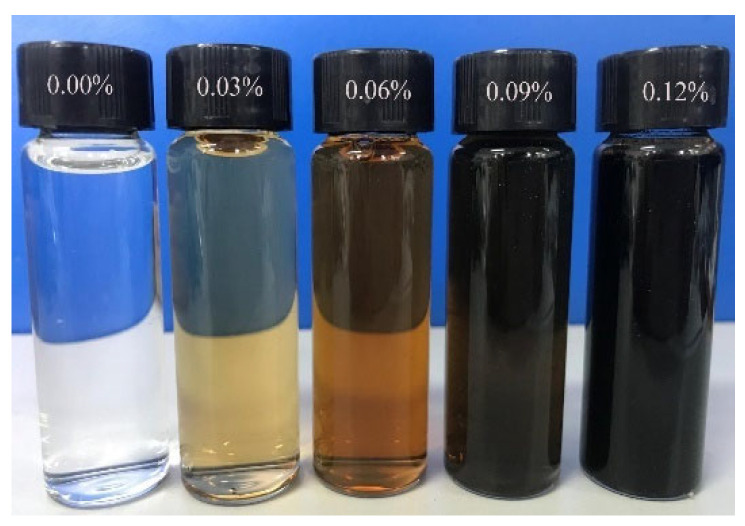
GO aqueous solutions after ultrasonic dispersion.
